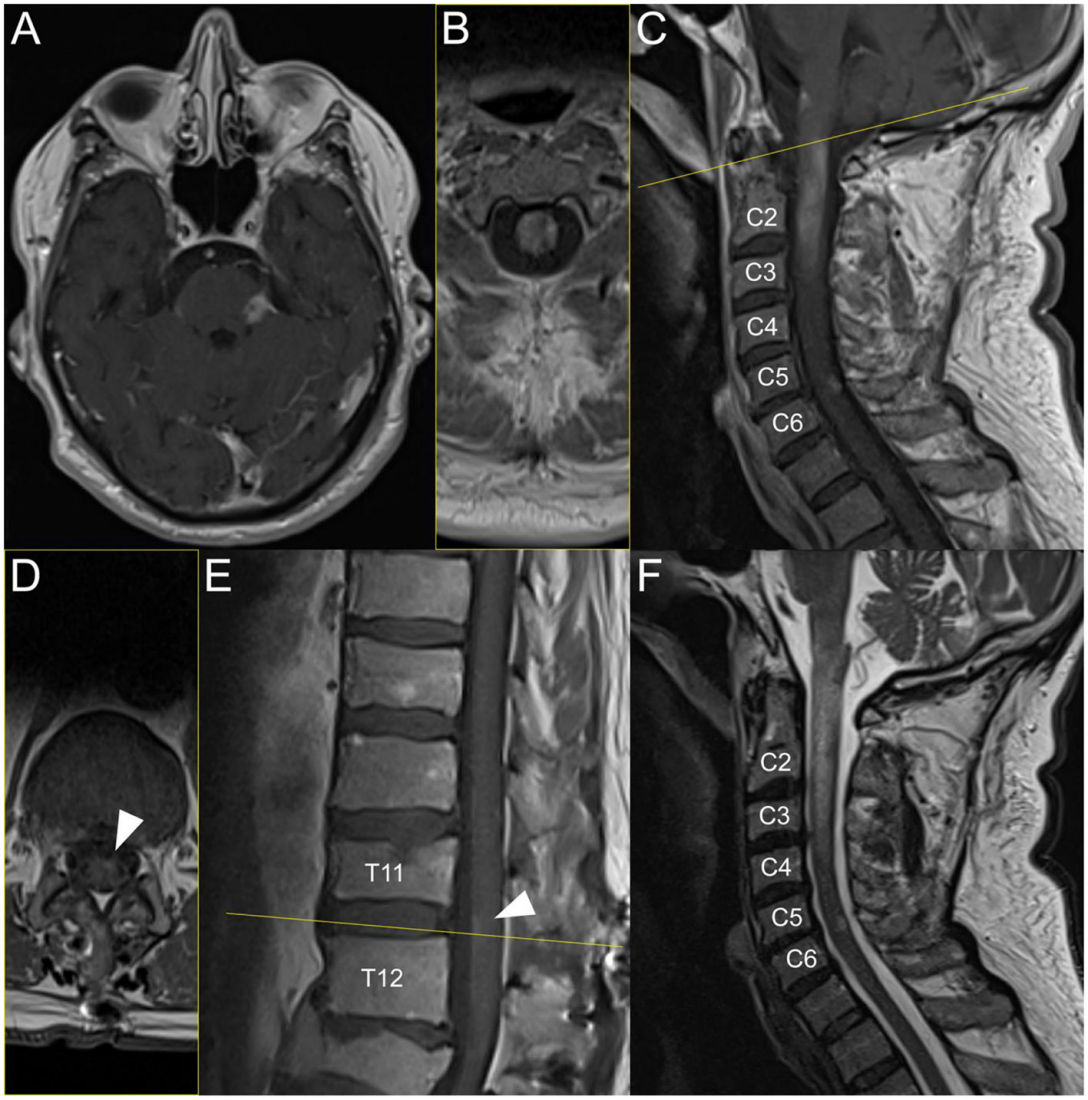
Brain and spinal MRI during post-SARS-CoV-2 infection-induced ADEM. Acute inflammatory contrast-enhancing T1 MRI lesions on the left middle cerebellar peduncle (A), longitudinal extensive transverse myelitis from the lower part of the medulla oblongata to C3 (C); [panel (B) shows axial plane, i.e., yellow line on panel (C)] with edema lesion on T2 (F) reaching C4, on level C5/C6 (C, F), and on the ventral conus (E); [panel (D) shows axial plane, i.e., yellow line on panel (E)].
